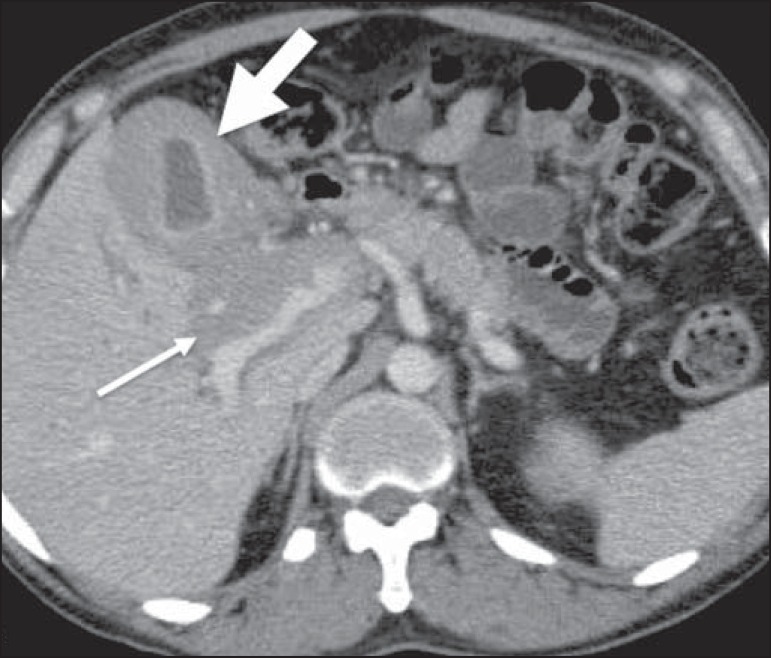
Gallbladder involvement by lymphoma. Axial CT of the abdomen, showing
